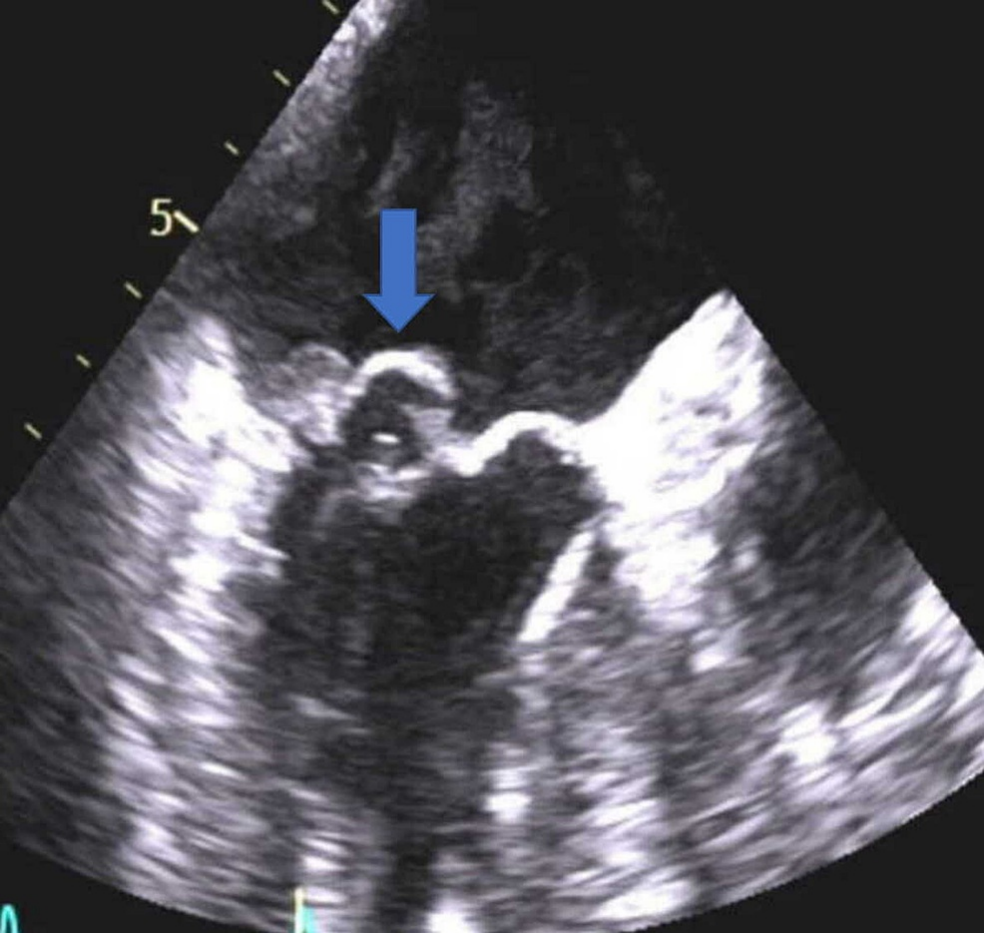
Transoesophageal echocardiogram showing mitral valve thickening and vegetation
